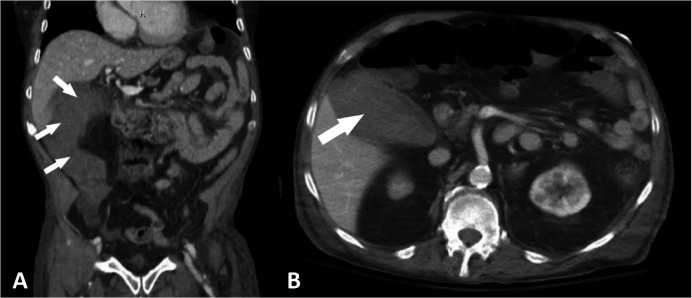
CT abdomen/pelvis demonstrating acute hemorrhagic cholecystitis with perforated/ruptured gallbladder with large heterogenous fluid collection fluid (arrows). Coronal (A) and axial (B) images.
